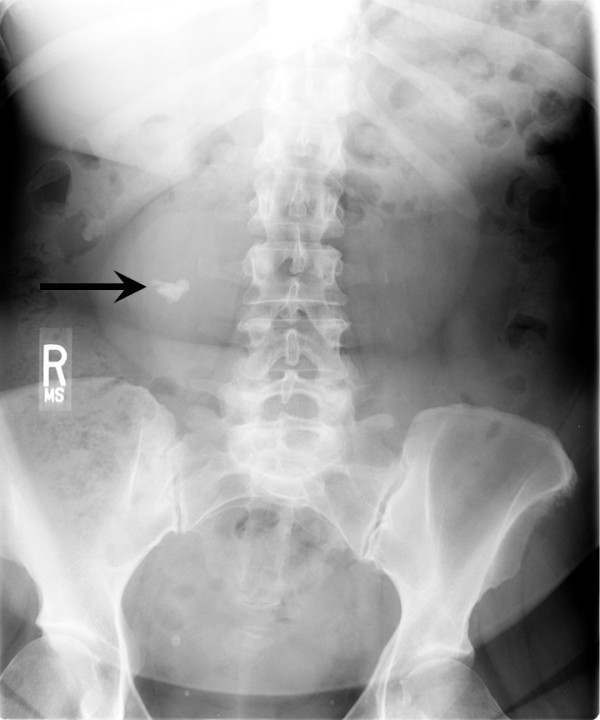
Anterior-posterior view of lumbar X-ray showing a large calcification (arrow) overlying the lower pole of the right kidney. R indicates right side.
